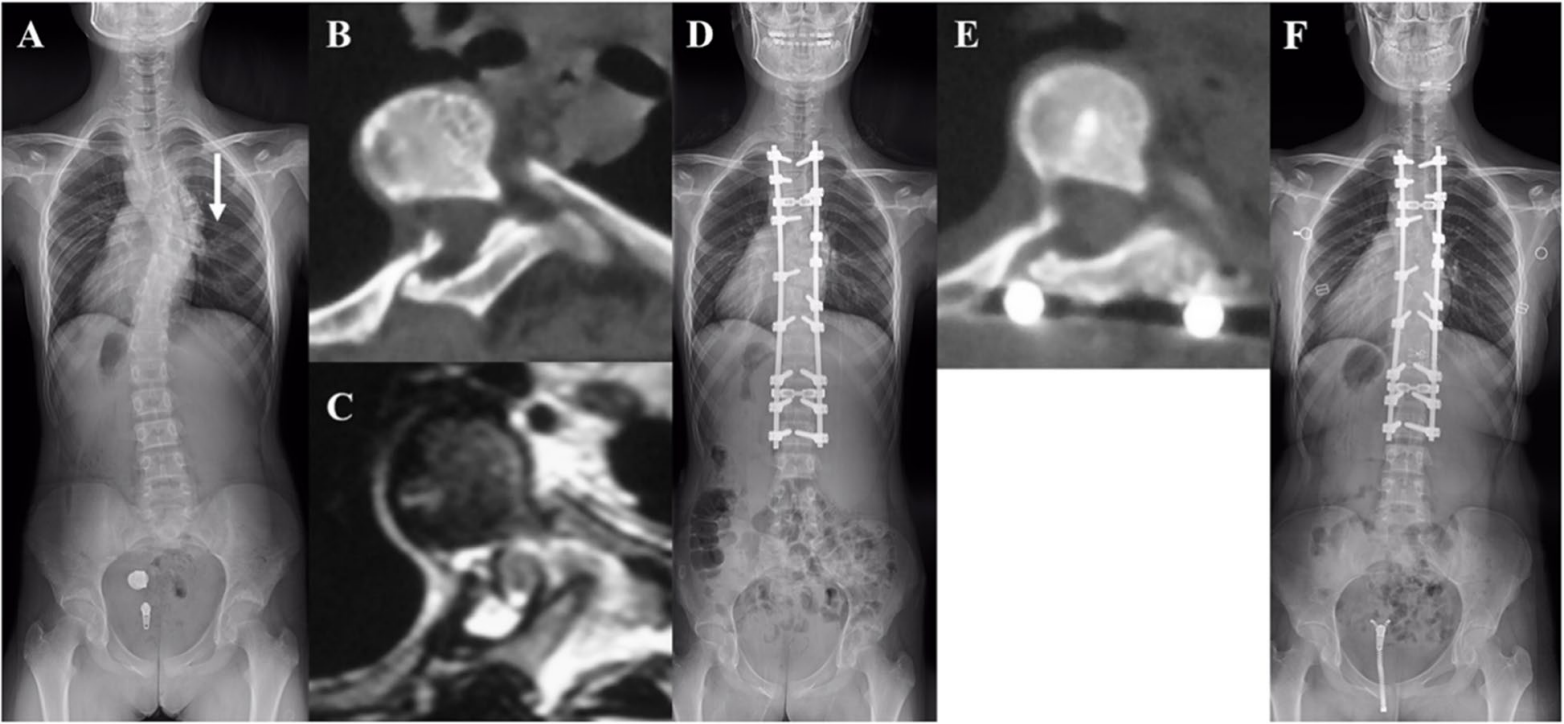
A 13-year-old girl with right thoracic dystrophic scoliosis secondary to NF-1 received surgical treatment in our institution. A The preoperative Cobb angle of thoracic curve was 55° with 7^th^ rib head dislocation and the vertebral translation (VT) of T7 was 28.5 mm B and C. The preoperative CT and MRI scans revealed the 7^th^ rib head penetrating the foramen and compressing the dural sac but not the spinal cord. The intraspinal rib length (IRL) reached 10.3 mm and the T7 showed a notable rib-vertebral angle (RVA) of 70°. D Posterior-only spinal fusion without rib head excision was performed, and pedicle screw was inserted at the level of dislocated rib. The Cobb angle was corrected to 24° postoperatively. E A good degree of rib head withdrawal was confirmed by post-operative CT scans. The IRL, RVA and VT were corrected to 2.8 mm, 57° and 13.5 mm postoperatively
